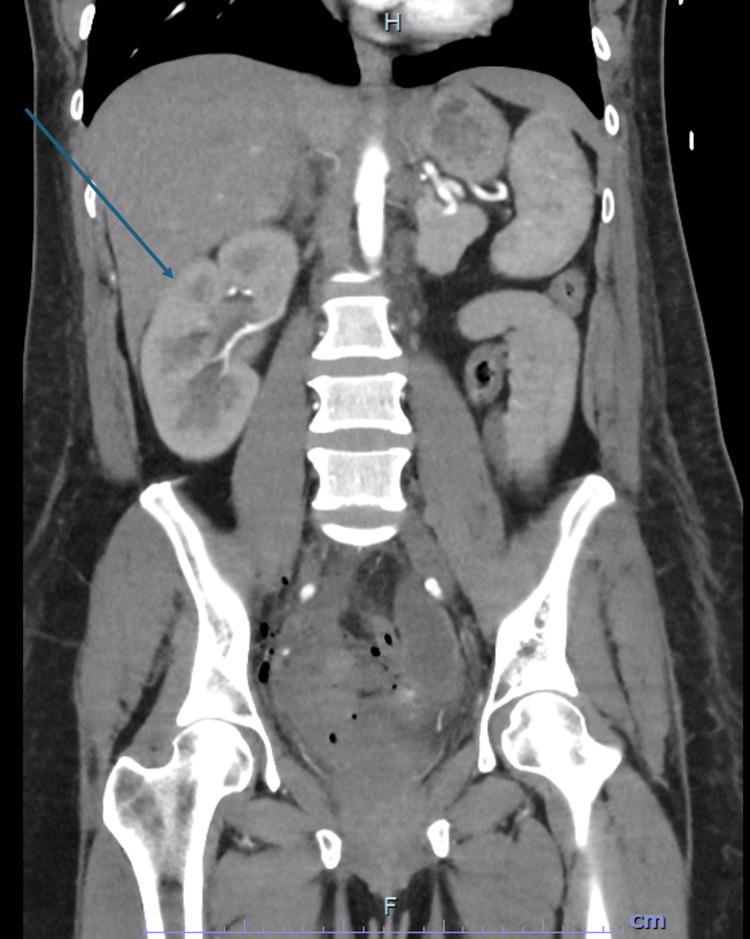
Coronal CT intravenous pyelogram demonstrating congenital absence of the left kidney and left renal artery, with compensatory hypertrophy of the right kidney (blue arrow). This confirmed the postoperative diagnosis of an associated renal tract anomaly and supported that the absent left ureteric orifice identified intraoperatively reflected congenital renal agenesis rather than iatrogenic ureteric injury.
